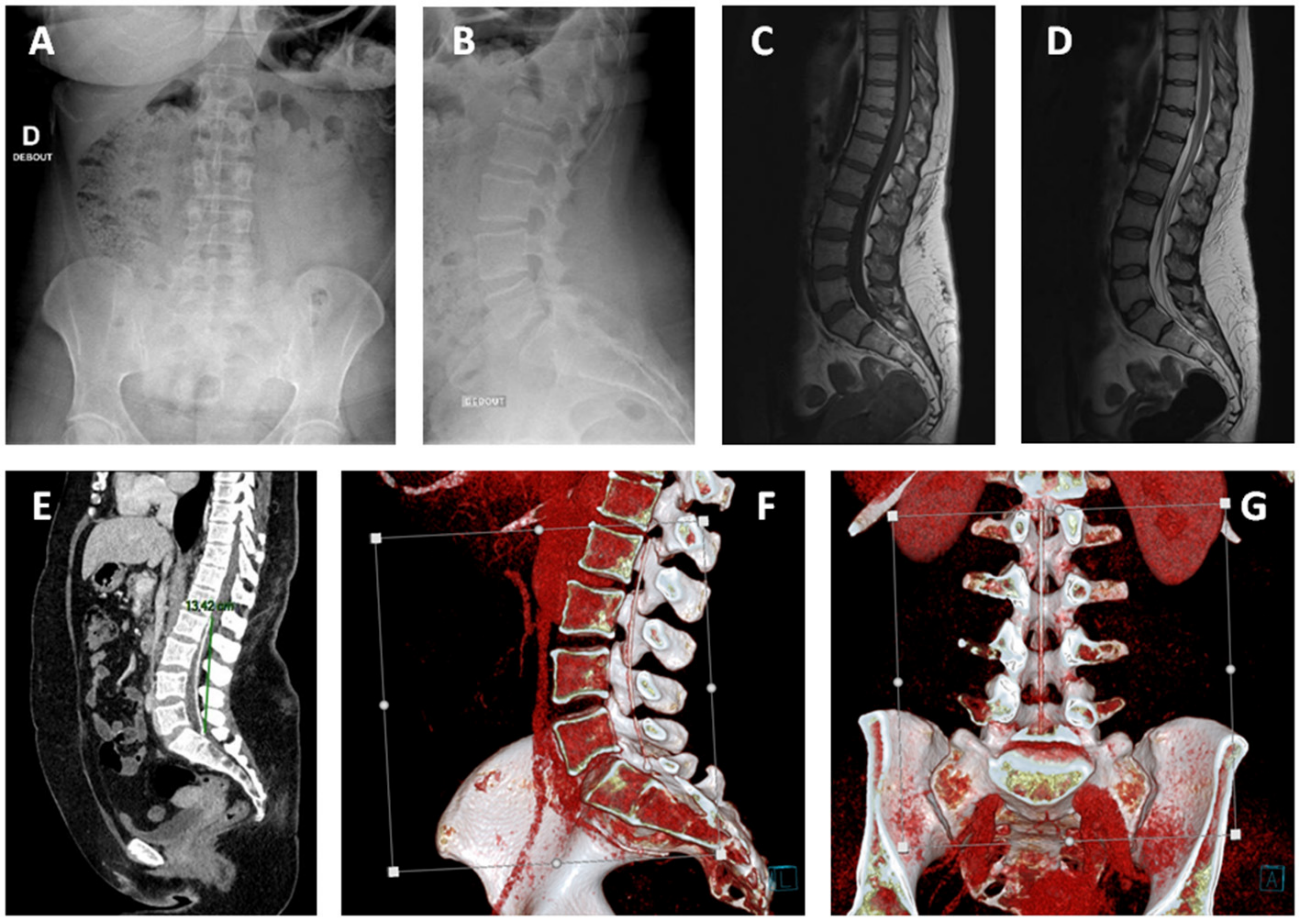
Lumbar x-rays: anteroposterior (A) and lateral views (B), MRI sagittal view, respectively, T1 (C), and T2 (D) sequences. The presence of the catheter was hard to identify even for experienced radiologists, as its intensity on T1-weighted and T2-weighted images was similar to that of the cauda equina nerve roots. (E) Lumbar CT scanner sagittal view showing the initially placed fourth ventricle to cervical subarachnoid space shunt located at L2-S1 level measuring around 15 cm in length and 2.5 mm in diameter. (F,G) Three-dimensional (3D) reconstructions. There is no local compression of neurological elements.
